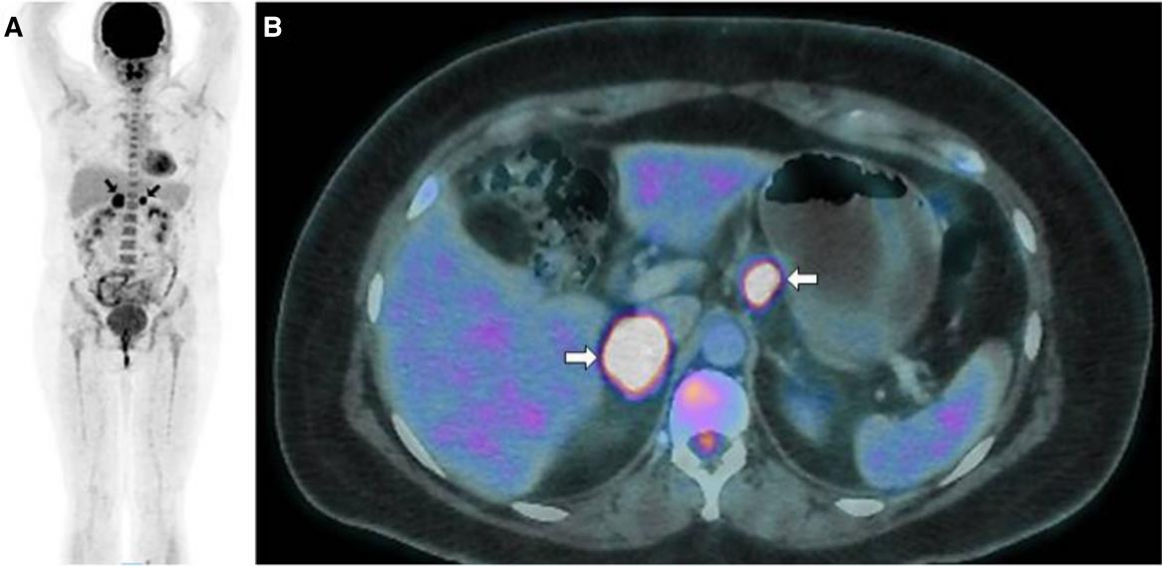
F-18 fluorodeoxyglucose positron emission tomography (18F-FDG PET/CT) image. A) Whole-body scan showing increased uptake in both the right adrenal mass and the solid nodular tissue in the hepatogastric space (dark arrows), but no lesions were observed in the liver or lungs. B) Axial slice from fused 18-FDG PET/CT showing that the right adrenal mass was markedly hypermetabolic, as was the nodular tissue in the hepatogastric space (white arrows), with standard uptake value (SUV) of 10.6 and 10.9, respectively.
